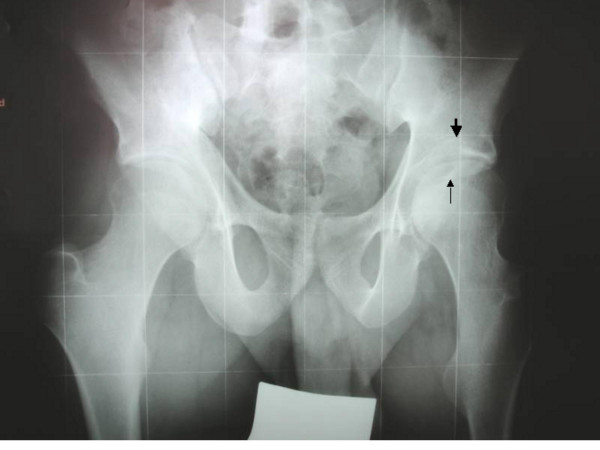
Patient 1: Pelvis Anteroposterior radiograph at age of 15 years showed coxa valga, relative flattening of capital femoral epiphyses, joint space narrowing and irregularity, with features of left acetabular osteochondritis associated with necrotic adjacent femoral epiphysis (arrows).
